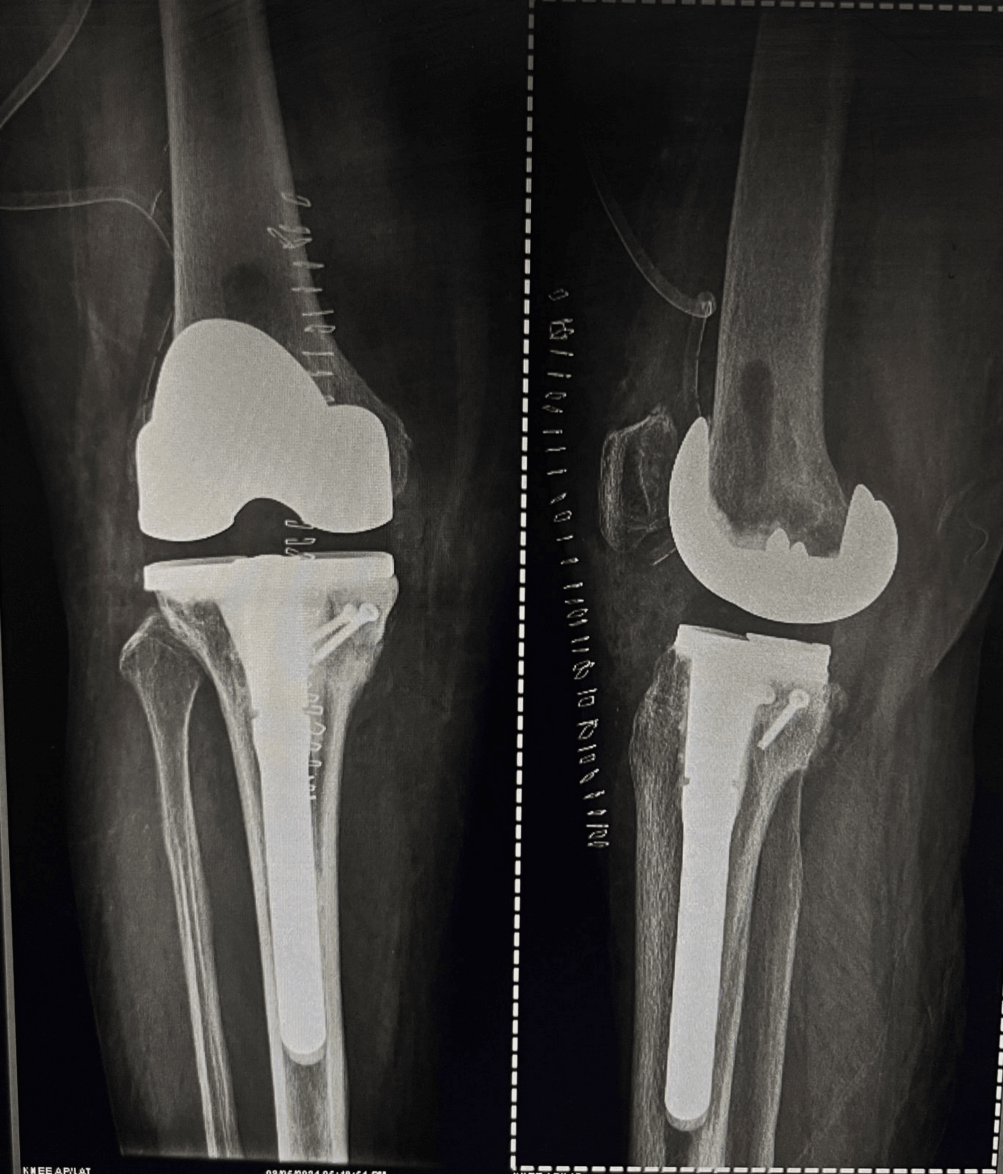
Postoperative X-ray. The extreme varus was corrected, osteophytes cleared on femoral and tibial side, posteromedial bone loss filled with cement and stabilized with screws.
